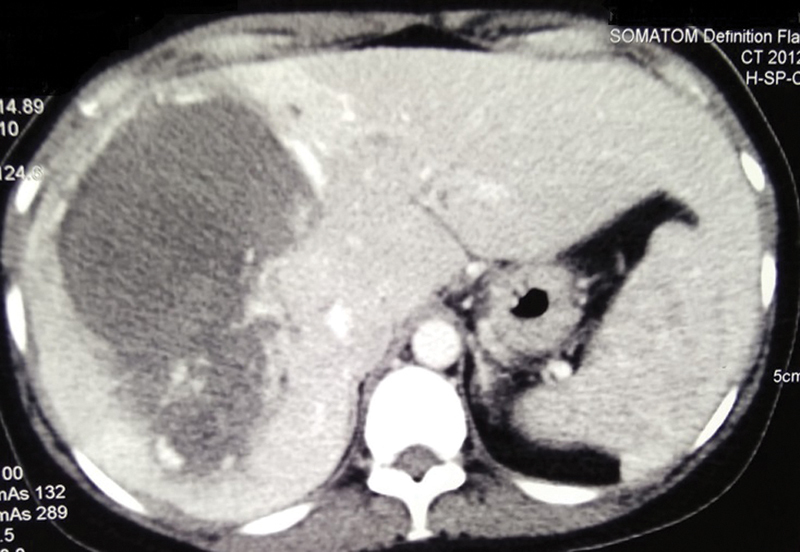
Computed tomography scan arterial phase depicting large peripherally enhancing lesion involving the right lobe of liver.
